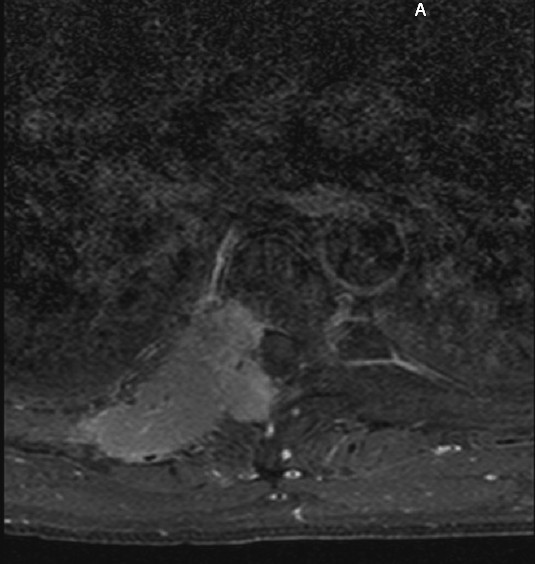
Contrast-enhanced T1-weighted axial MRI of the T6 level for patient #2. This slice demonstrates a large multi-lobulated mass involving the vertebral body and neural arch with extension into the spinal canal, intervertebral foramen and chest wall. It represents one focus of metastases from a primary renal cell carcinoma.
